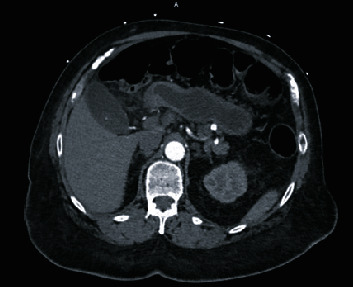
Preoperative CT scan shows mild distention of the gallbladder with gallstones and adjacent inflammatory stranding extending to the first and second portion of the duodenum.
